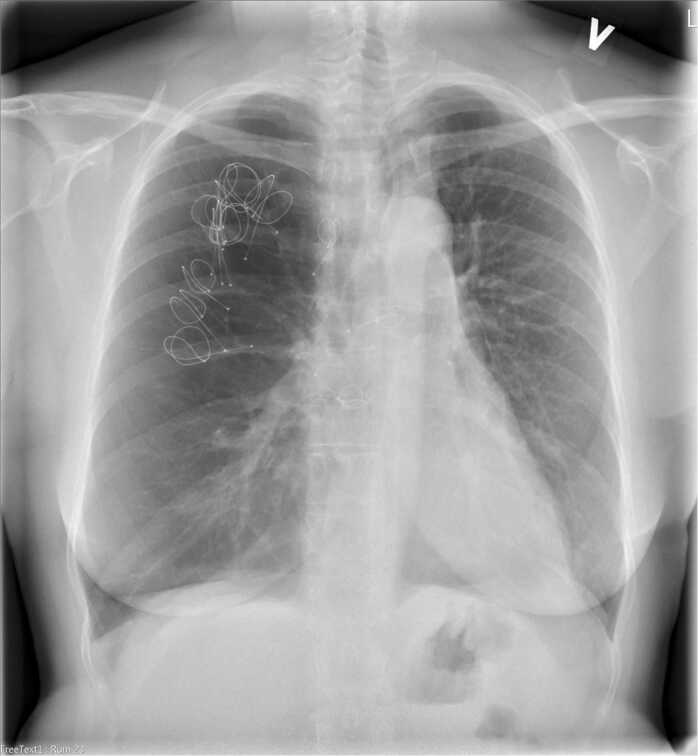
Postoperative chest X-ray after first LVRC treatment of right upper and middle lobes. LVRC, lung volume reduction coils.
